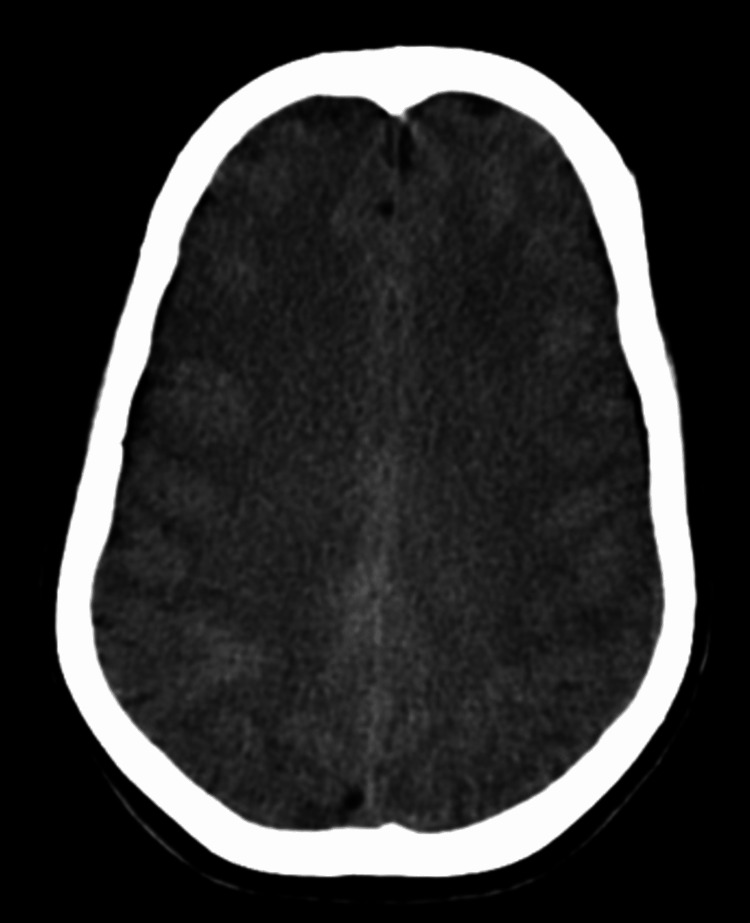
Non-contrast CT of the brain showing diffuse cerebral edema.
